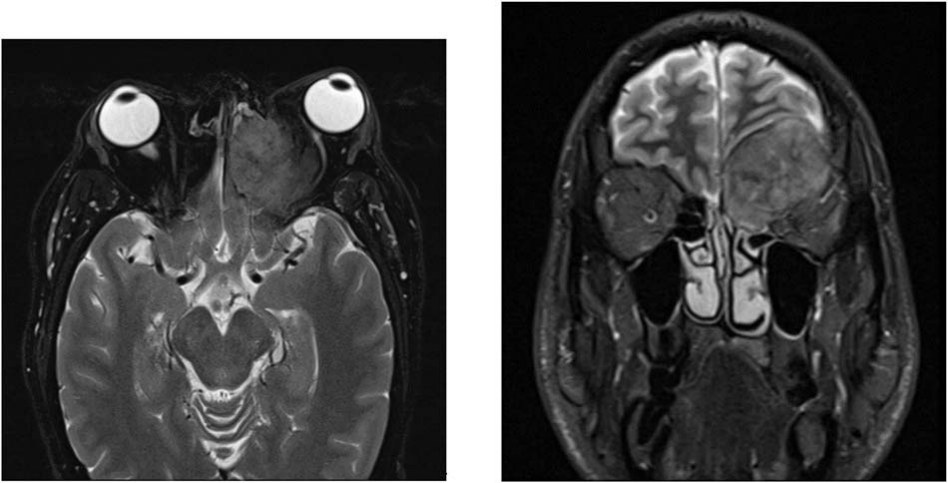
MRI with contrast shows a large left anterior cranial fossa and extra-axial extradural solid mass involving the left olfactory groove with bony erosion. There is a large component on the superior and medial extraconal orbital causing left proptosis. Direct involvement of the left frontal sinus is observed. (a) Axial view. (b) Coronal view.
